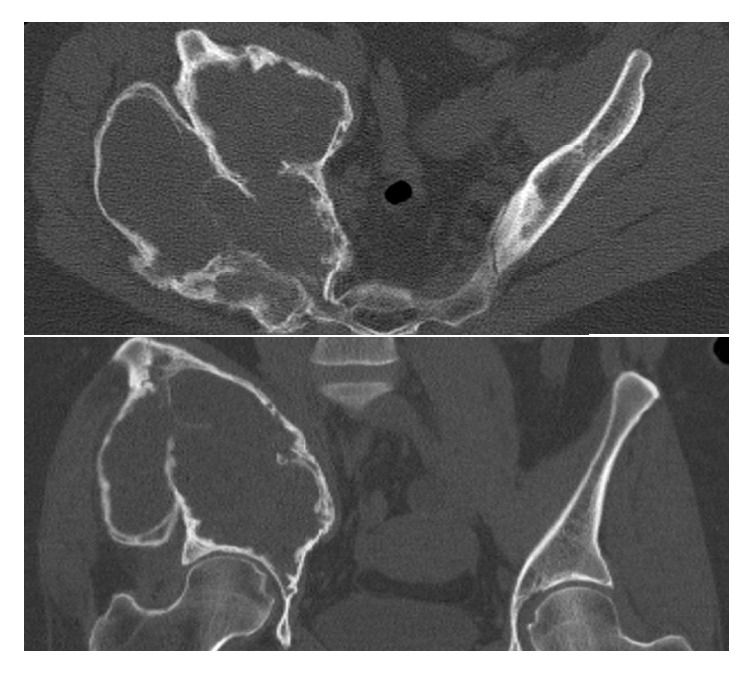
Preoperative follow-up CT scan showing a notable increase in cortical thickness.
